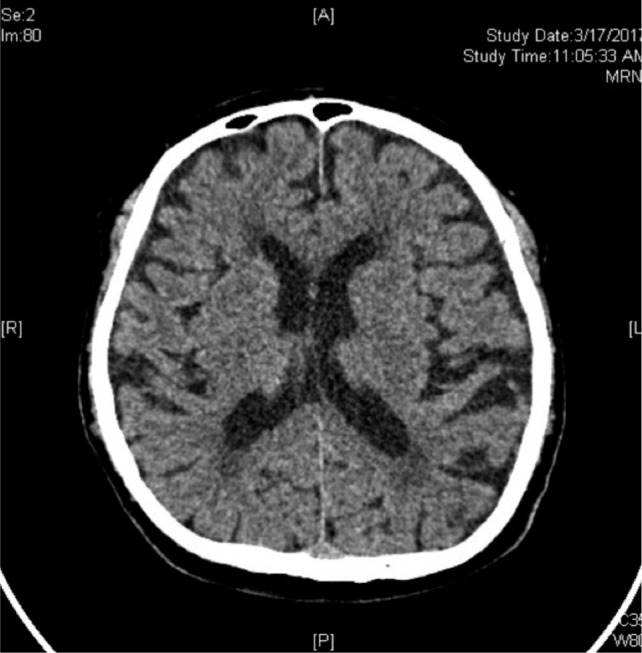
CT Brain repeated 3 days after the seizure showing complete resolution of the air pocket.
